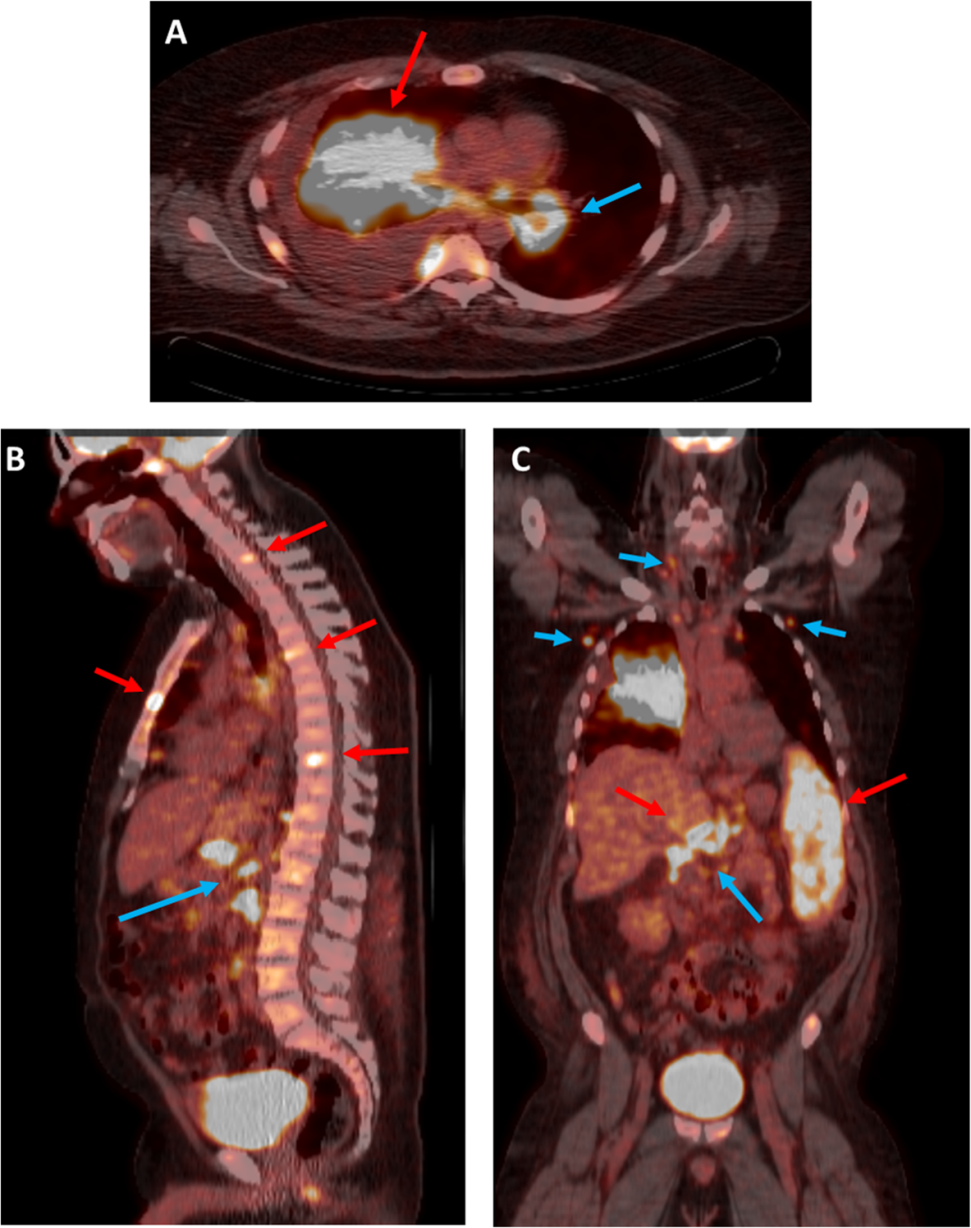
Axial, sagittal and coronal fused PET-CT images demonstrating widespread radiotracer uptake, including within right lung mass (red arrow in a), liver, spleen, multiple bones (red arrows in b and c) and multiple lymph node stations in lower neck, chest and upper abdomen (blue arrows in a, b and c)
